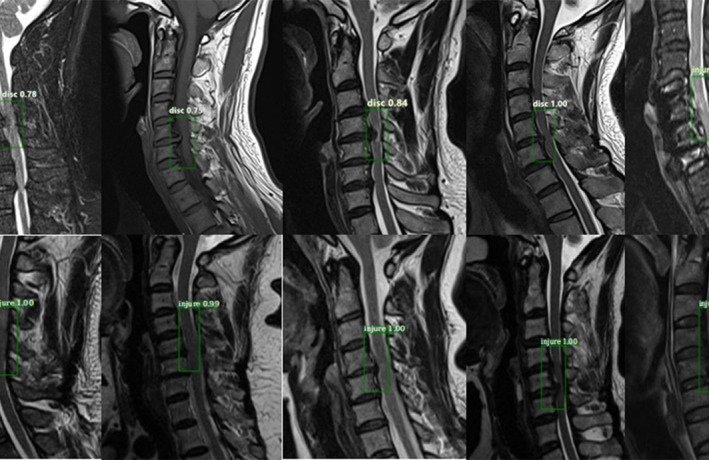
Example of detection results: from the outputs, each picture is surrounded by a target area and the corresponding classification name and predicted probability value. This is an efficient method to recognize the effects of lesions on magnetic resonance imaging images. The experimental results are observed to have reached the expected goal.
